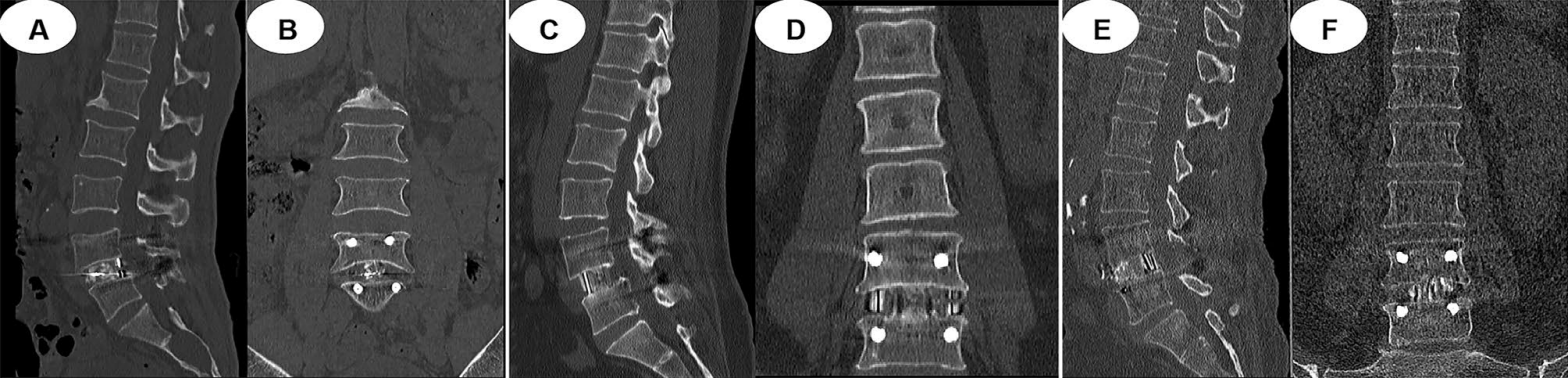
(A, B) CT at 12 months postoperatively showing intervertebral fusion after MIS-TLIF. (C, D) CT at 12 months postoperatively showing intervertebral fusion after OLIF. (E, F) CT at 12 months postoperatively showing intervertebral fusion after Endo-TLIF.
